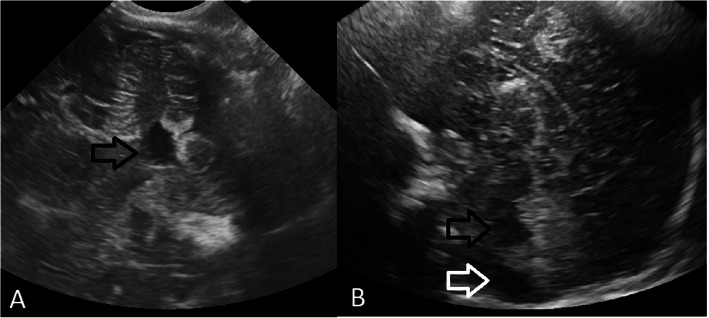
Brain ultrasound. a. Axial view through the mastoid fontanel: enlarged fourth ventricle (black arrow). b. Sagittal view through the anterior fontanel: cerebellar vermis hypoplasia with secondary enlarged of the fourth ventricle (black arrow) and enlarged *cisterna magna* (white arrow)
